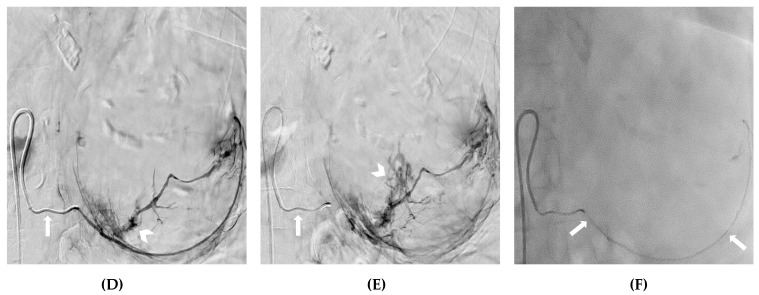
(A) A CTA showing spontaneous retroperitoneal bleeding due to an unknown, ruptured, adrenal myelolipoma. (B) A digital subtraction angiography depicting the inferior adrenal artery arising (arrow) from the proximal left renal artery, along with the shadow (arrowheads) of the ruptured myelolipoma displacing the left kidney downwards. (C,D,E) The superselective catheterization of the target artery with a microcatheter (arrow) and digital subtraction angiography, showing a massive intratumoral hemorrhage (arrowheads). (F) A fluoroscopy demonstrating the embolization of the inferior adrenal artery, using PVA particles mixed with iodinated contrast media (arrows).
